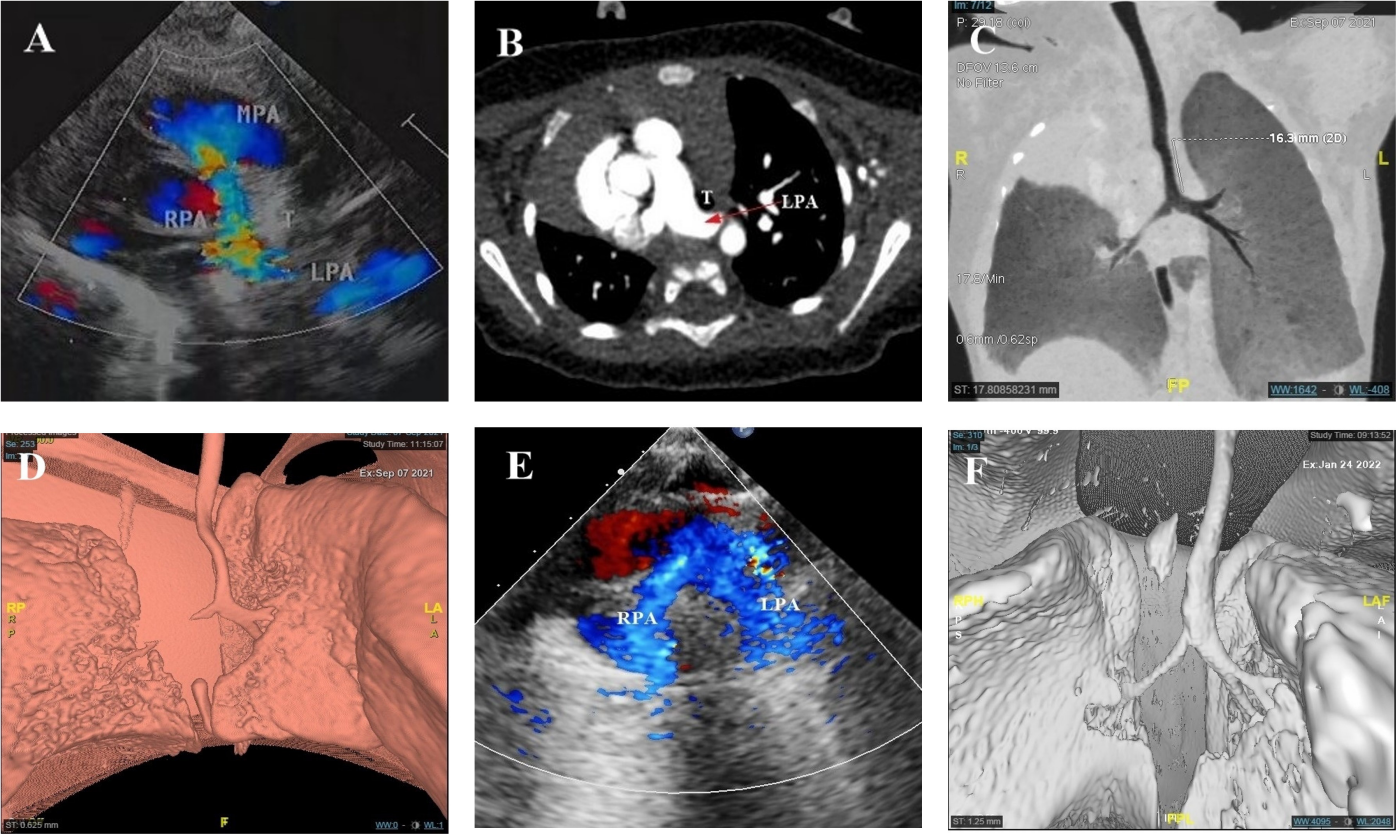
Integrated prenatal and postnatal management of PAS (postpartum portion). (A,B) Neonatal echocardiography and CTA showed PAS. (B–D) Minimum density projection and 3D tracheobronchial reconstruction reported long-term stenosis in the middle and upper long segment of the airway, the stenotic lower trachea, and the stenotic trachea above the carina. (E) Postoperative echocardiography showed that the left and right pulmonary arteries originated from the main pulmonary artery. (F) 3D tracheobronchial reconstruction indicated a significant improvement in tracheal stenosis when compared with the preoperative period.
